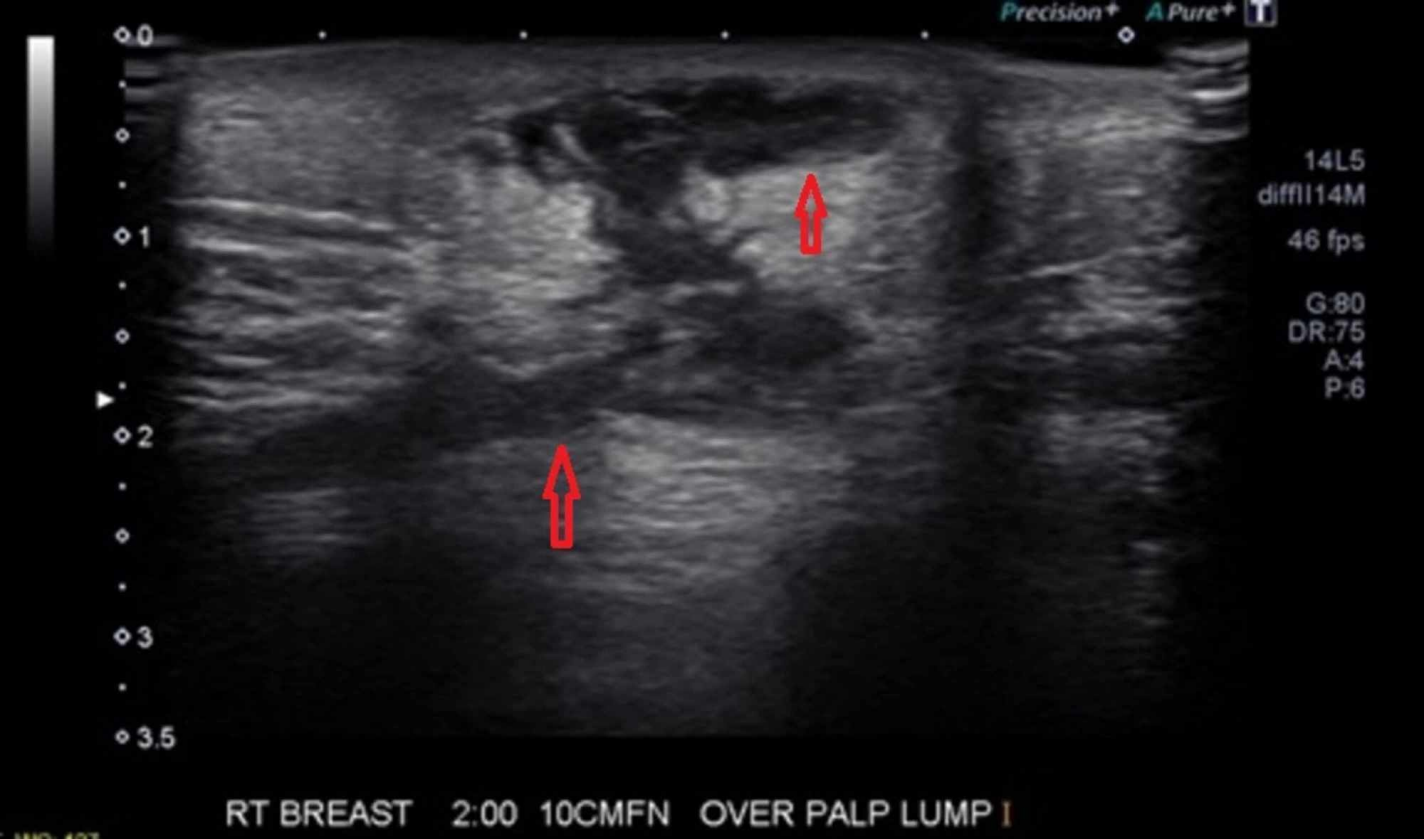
Breast ultrasound Breast ultrasound (US) before incision and drainage (I&D) shows complex partially fluid-filled parenchymal changes directly under the indurated area (upper red arrow) with extension into the biopsied granulomatous mastitis lesion suspicious for fistularization with possible abscess or phlegmon formation (lower red arrow).
